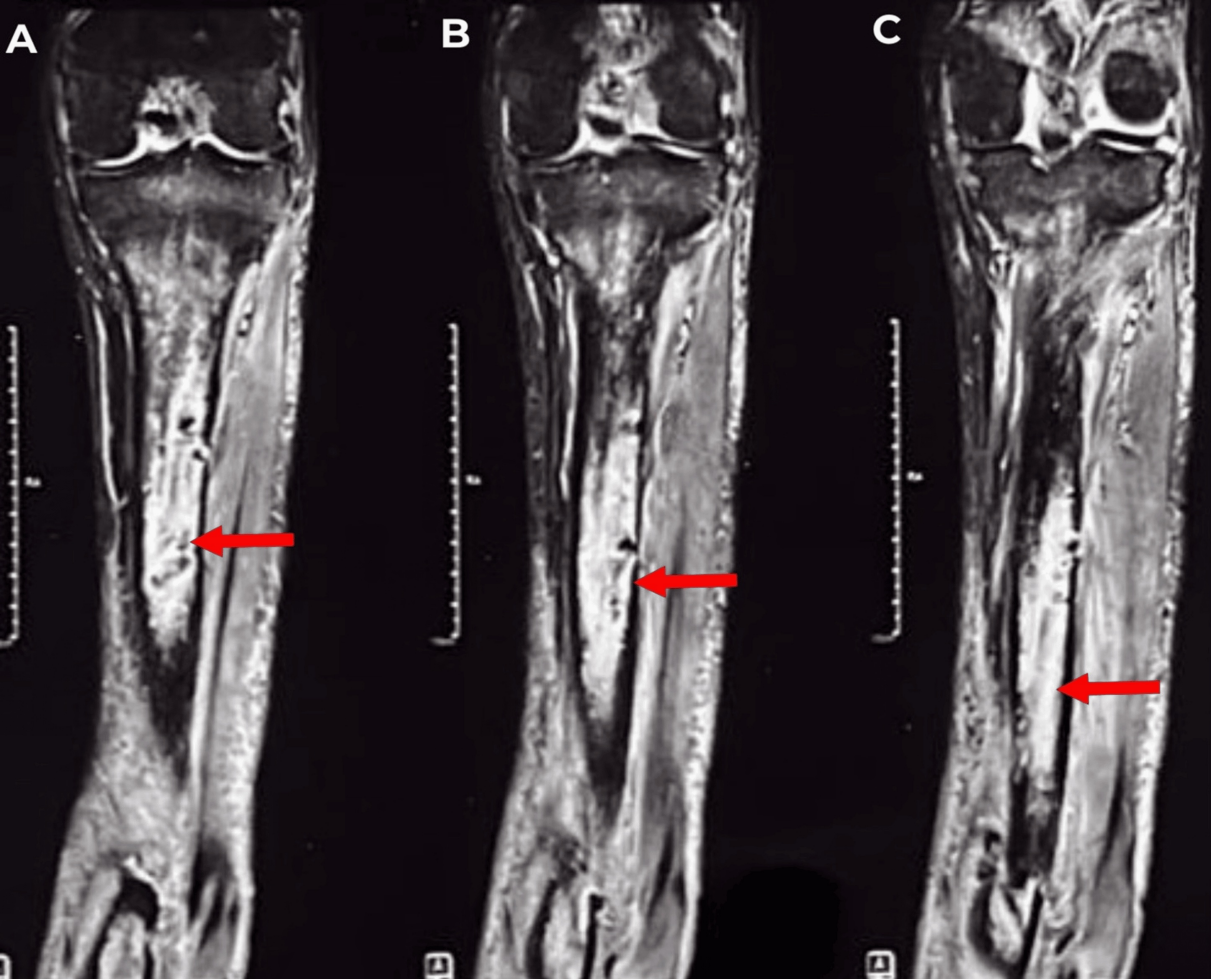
A-C) Coronal sections of the left leg MRI. Red arrows show fluid collection in the medullary canal of the tibia. MRI: magnetic resonance imaging
